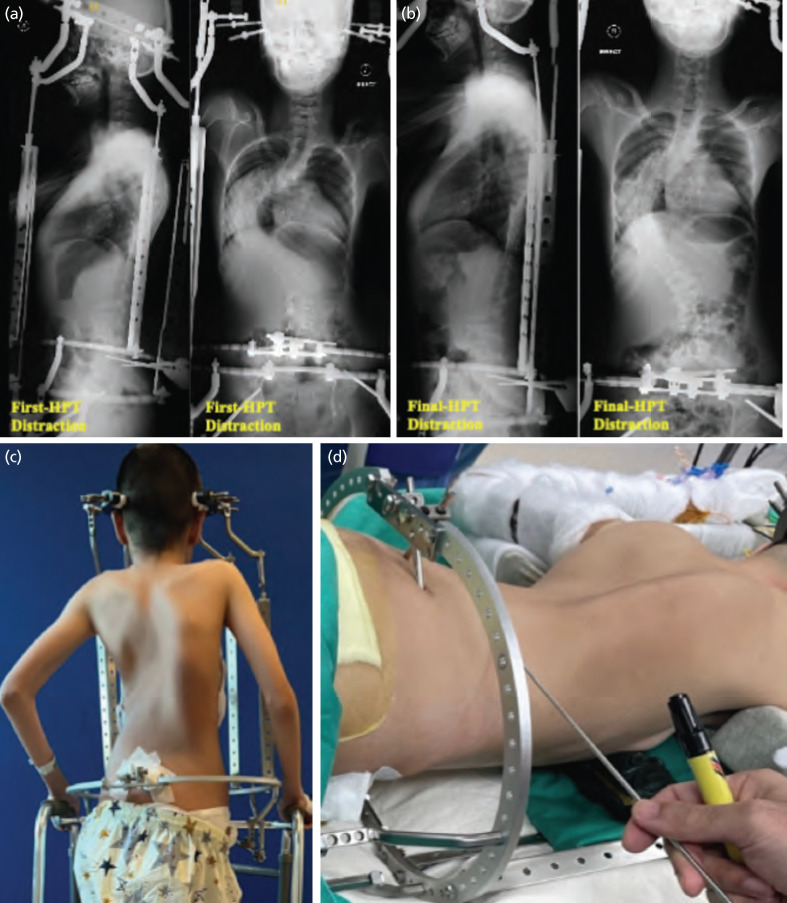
(a) Anterior-posterior and lateral views of the erect spine plain radiograph shows a Cobb angle improvement of 38° and a sagittal angle improvement of 16° with the first HPT distraction. (b) After three distractions, there was a marked improvement in the kyphotic angle (from 90° to 64°) and the Cobb angle (from 158° to 103°) with HPT. (c) Two posterior struts were shifted to the anterior for definitive spinal surgery. (d) Posterior spinal fusion done with halo pelvic frame in situ and loosened struts.
